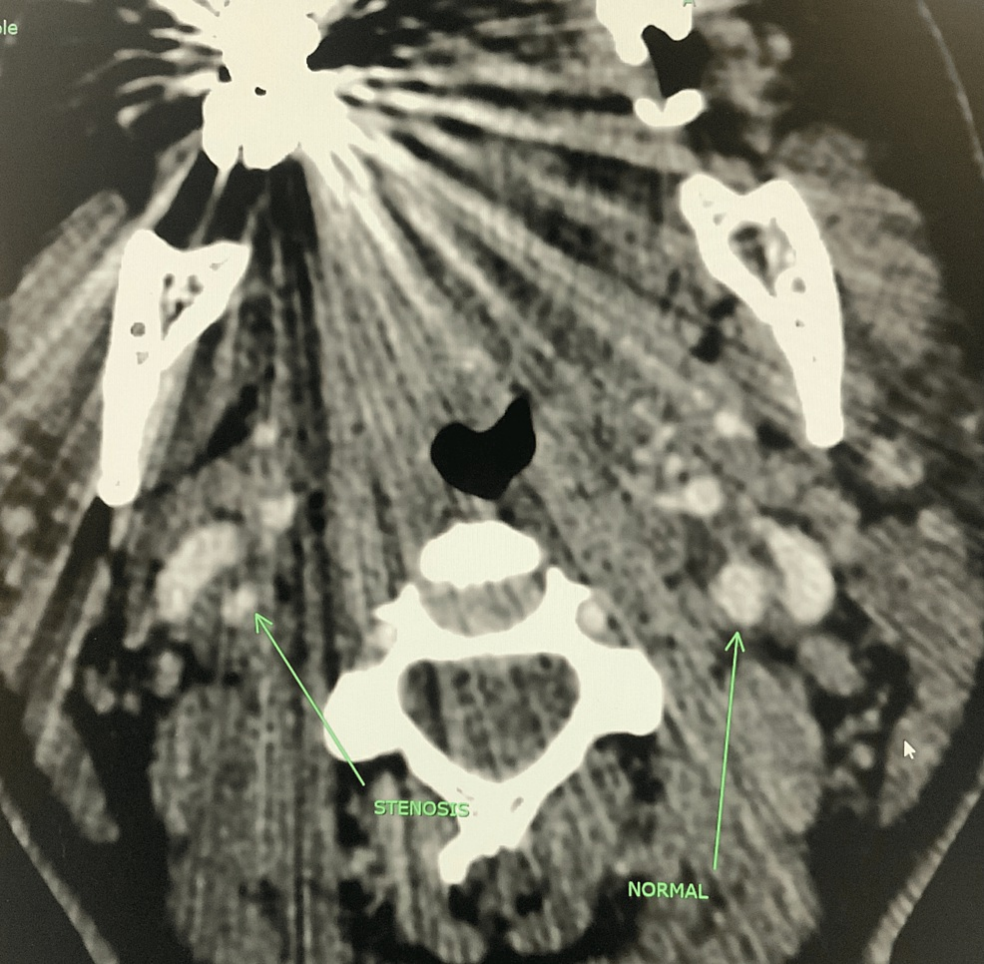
CT of the head showing right-sided (>70%) carotid stenosis and thrombosis (arrow) CT: computed tomography
